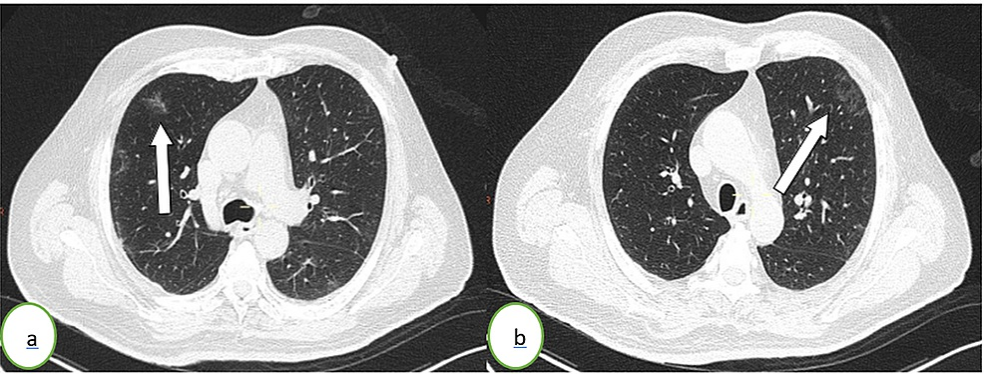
Thoracic CT scan in an axial (a, b) lung window showing subpleural ground-glass opacities (white arrows) in the left upper lung lobe The percentage of lung involvement is <10%.
